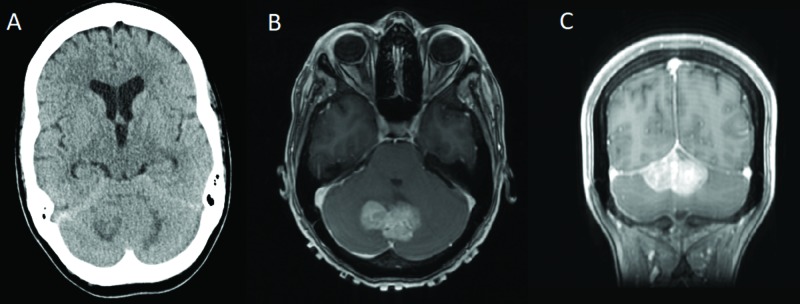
Preoperative imaging. A) Axial CT without contrast demonstrating a midline, superior cerebellar lesion with early hydrocephalus; B) Axial T1 MRI with contrast demonstrating lobulated, enhancing, likely extra-axial mass with low signal on T1; C) Coronal T1 MRI with contrast demonstrating lobulated, enhancing, likely extra-axial mass with low signal on T1 Figure 3A and Figure 3B republished with permission from Chica J, Yepes I, Burks SS, Komotar R, Carrillo R. Case of an intracranial malignant peripheral nerve sheath tumor in the setting of pacer-dependent heart block. Asian Journal of Neurosurgery, Volume 13, Issue: 1, Pages 147-149. Wolters Kluwer- Medknow CT: computed tomography; MRI: magnetic resonance imaging
